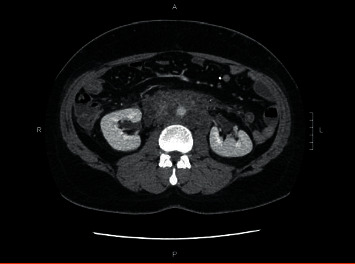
CT imaging of the abdomen and pelvis reveals large retroperitoneal process with dense infiltrative tissue extending along the aorta at day 9.
